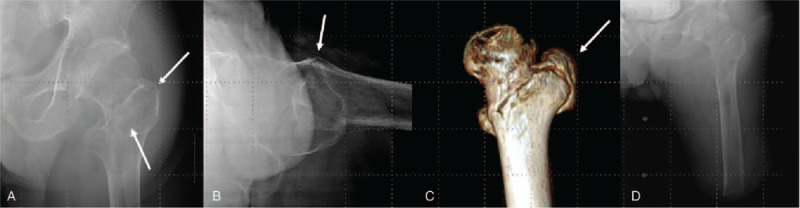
Case 2. (A) and (B) Preoperative plain radiograph images showing an intertrochanteric fracture in an 89-year-old patient with above-knee amputation (thin white arrow). (C) Three-dimensional computed tomography image showing a left intertrochanteric hip fracture. (D) Anteroposterior radiographs showing the above-knee amputation.
